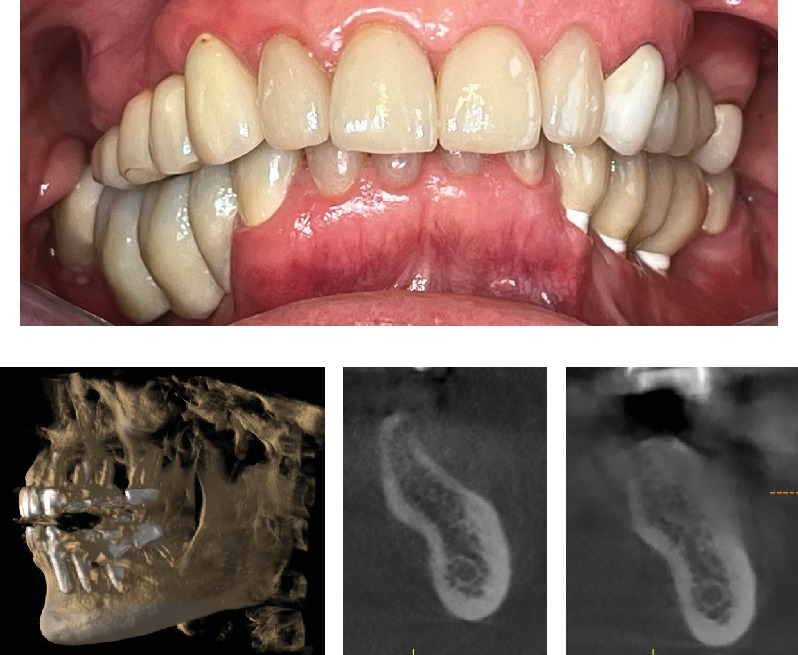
One-year follow-up after implant placement. (a) Frontal view clinical photograph, (b) CBCT showing distortion due to the presence of artifacts caused by the ceramic implants, (c) CBCT cross-sectional view of defect site prior to augmentation, and (d) CBCT cross-sectional view of defect at 1-year follow-up.
